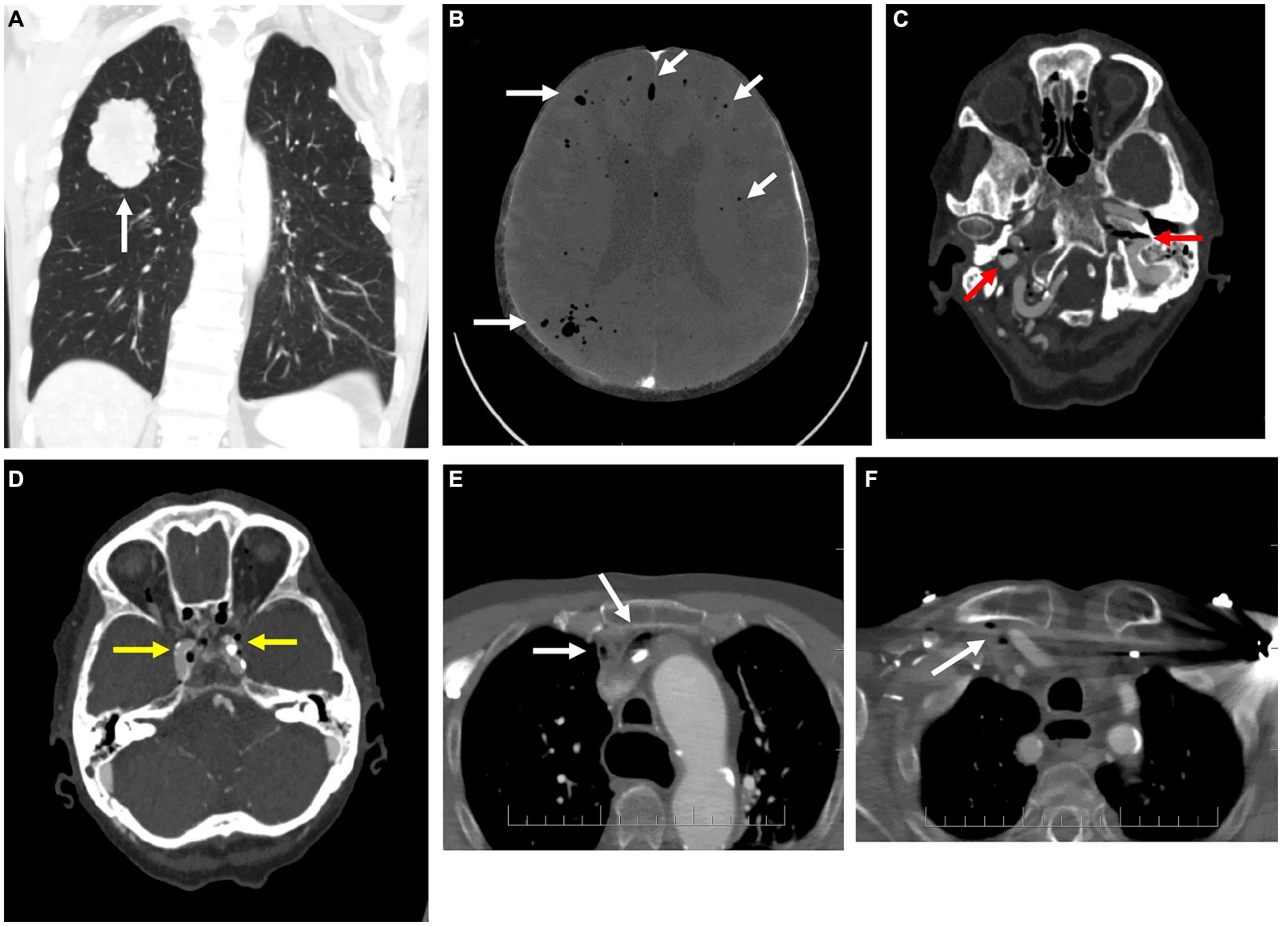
Imaging for cases 1 (A,B) and 2 (C–F). (A) Coronal plane of the native CT of the lungs showing a typical image of a primary lung tumor in the upper right lobe. (B) Axial CT scan of the brain in Minimum intensity projection (MinIP) shows the number of air bubbles in the cerebral circulation (white arrows, MinIP is an imaging technique used to identify low-density structures within a specific volume). (C,D) Axial contrast-enhanced CT scans of the brain with gas bubbles in the internal jugular veins (red arrows) and cavernous sinuses bilaterally (yellow arrows). (E,F) Axial contrast-enhanced CT scans of the upper thorax with air bubbles in the right subclavian vein and right and left brachiocephalic veins (white arrows).
